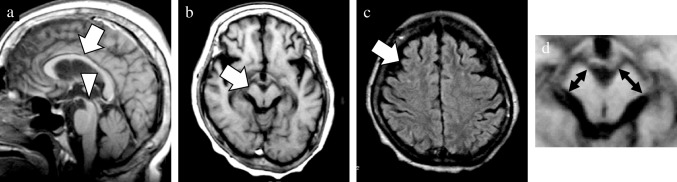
A representative case of radiologically atypical CBD. This 67-year-old female patient obtained an antemortem diagnosis of CBD. MRI was performed four years after the symptom onset. In addition to atrophy of the callosal body (arrow, a), mild midbrain atrophy was also detected on sagittal T1WI (arrowhead, b). In contrast to right dominant asymmetric atrophy of the cerebral peduncle and frontal lobe (arrows, b, c), no obvious SWMH was noted on axial FLAIR image (c). A magnified view was exhibited to emphasize the asymmetry of the short axis in the cerebral peduncles (double sided arrows, d). a, sagittal T1WI; b, axial T1WI; c, axial FLAIR image; CBD, corticobasal degeneration; FLAIR, fluid attenuated inversion recovery; SWMH, subcortical white matter hyperintensity; T1WI, T1-weighted image
